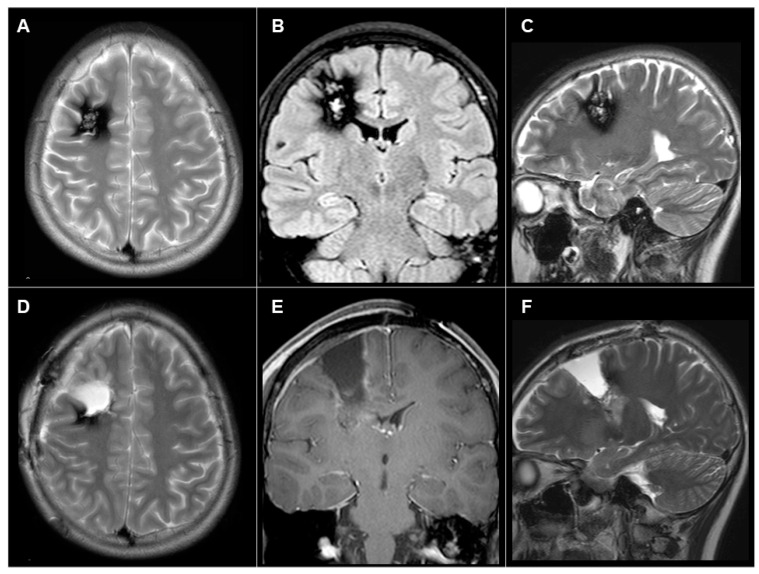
(A–C) Preoperative axial (A), coronal (B), and sagittal (C) magnetic resonance imaging (MRI) showing the cavernous angioma and surrounding hemosiderin deposit in the right frontal lobe. (D–F) Postoperative axial (D), coronal (E), and sagittal (F) MRI showing the removal of the cavernous angioma, with some parts of the hemosiderin rim remaining at the posterior edge.
